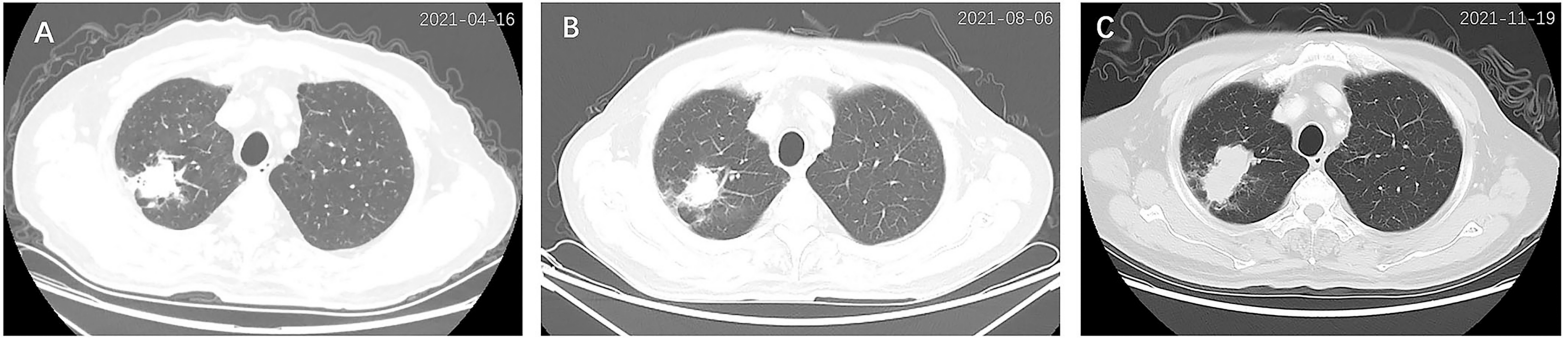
Chest computed tomography (CT) scan of the primary lung cancer at diagnosis (A), 3 months after treatment (B), and 6 months after treatment (C). An irregular mass (3.5 cm × 2.7 cm) was detected in the right upper lobe (A), which shrunk (2.6 cm × 2.2 cm) after 3 months of treatment (B) but enlarged (5.2 cm × 2.6 cm) after 6 months of treatment (C).
